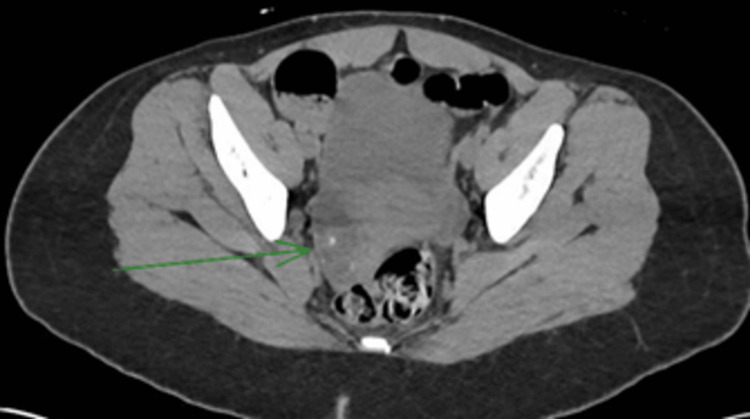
Axial CT scan showing a 6.1 cm right ovarian teratoma (green arrow). CT: Computed tomography
